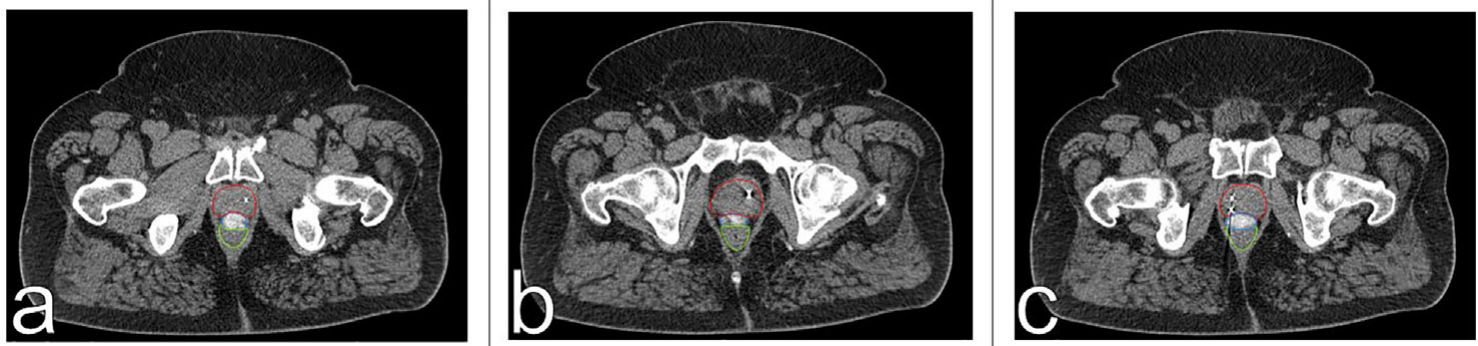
Axial CT images of spacer positioning at prostatic base (A), mid-gland (B) and apex (C). The spacer provides 1.1 cm (A), 1.65 cm (B), and 1.55 cm (C) of rectal separation. The prostate is contoured in red, the spacer in blue, and the rectum in green.
